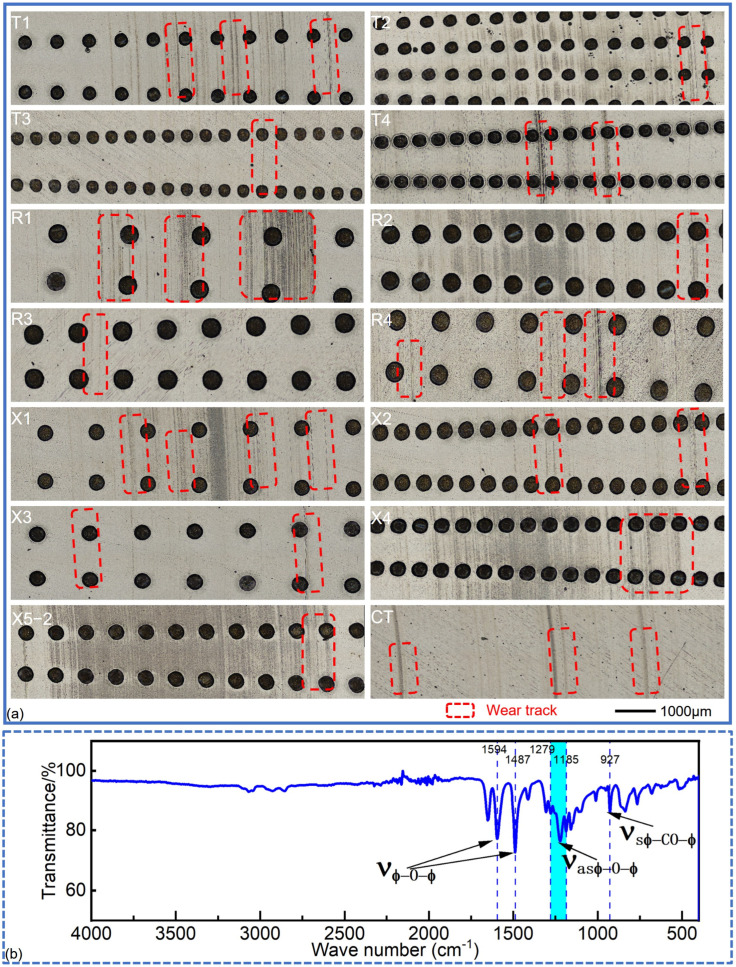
Representative wear morphologies of the 1040 steel discs after ultrasonic washing and the characteristic infrared absorption peaks of the PEEK transfer film: (a) wear morphologies of the 1040 steel discs; (b) typical infrared spectral characteristics of the PEEK collected on the surface of 1040 steel discs.
